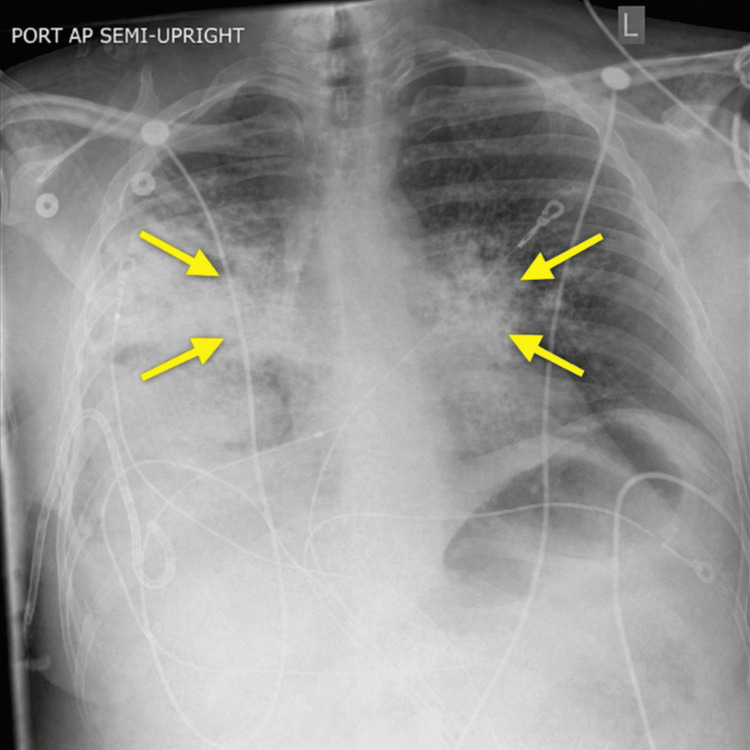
Chest X-ray consistent with re-expansion pulmonary edema Chest imaging demonstrating bilateral perihilar airspace opacities consistent with pulmonary edema, with yellow arrows highlighting areas of alveolar opacification.
